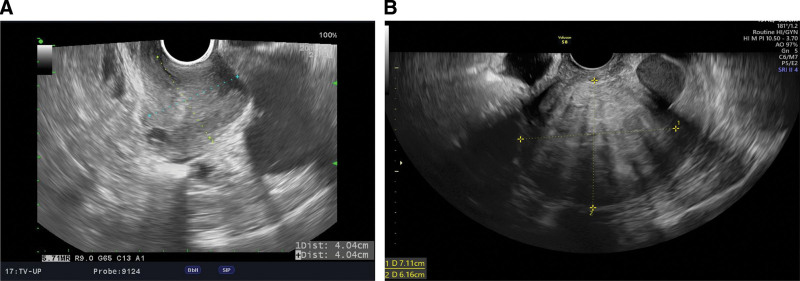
Pelvic ultrasound images of the cervix after supracervical hysterectomy. (A) Remnant cervix after supracervical hysterectomy. (B) Cervical adenomyoma developed 7 years after the first surgery.
